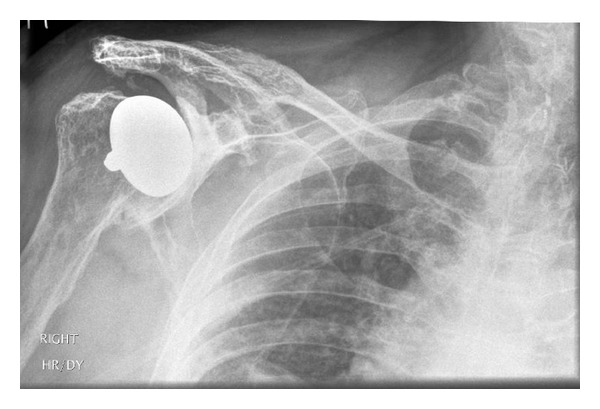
AP radiograph of the right shoulder taken 4 years postoperatively.
